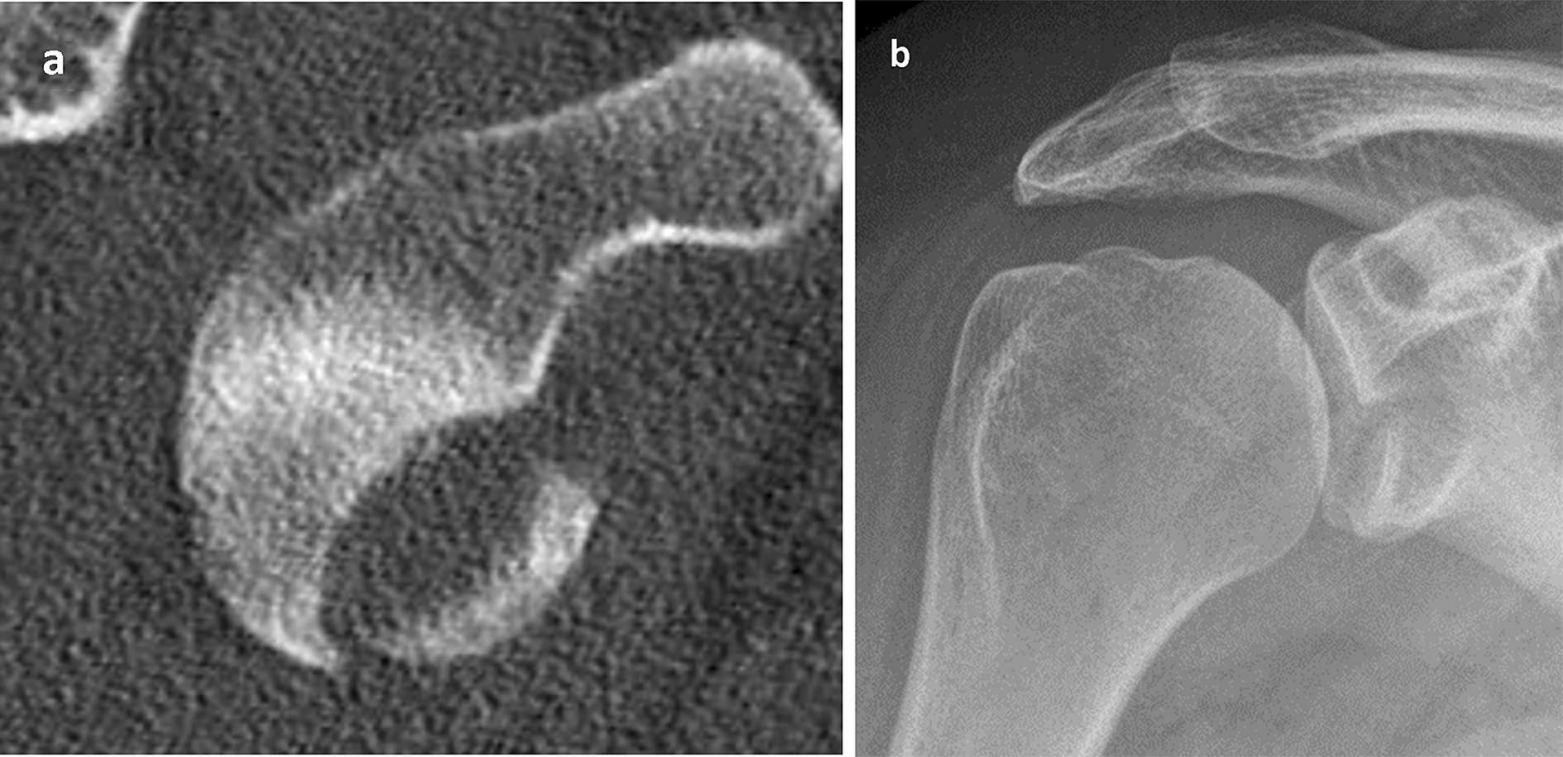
Concentrically reduced humeral head in the A.P.-radiograph is the main prerequisite for nonoperative treatment of GRF and represents the main criterion within radiographic controls (b CT en face view; a A.P.)
